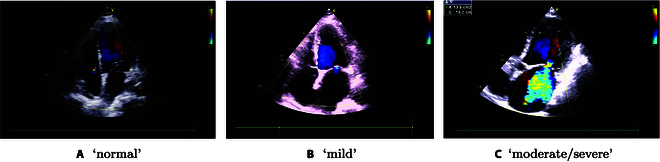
The echocardiography samples of the participants. (A) There were signals of regurgitation detected of the mitral valve orifice from the apical 4-chamber section. (B) There were color Doppler and a small amount of mitral regurgitation signals detected from the apical 4-chamber section. (C) There were mitral valve orifice and a lot of regurgitation signals detected from the apical 5-chamber section.
